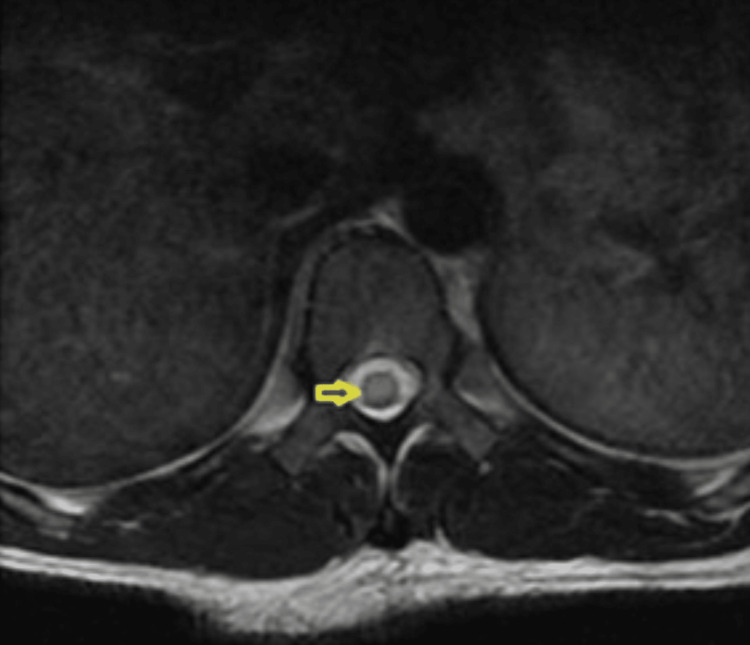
Repeat thoracic spine MRI on hospital day four with contrast enhancement showing an intramedullary T2 hyperintense signal and expansion at the lower thoracic cord/conus medullaris.
